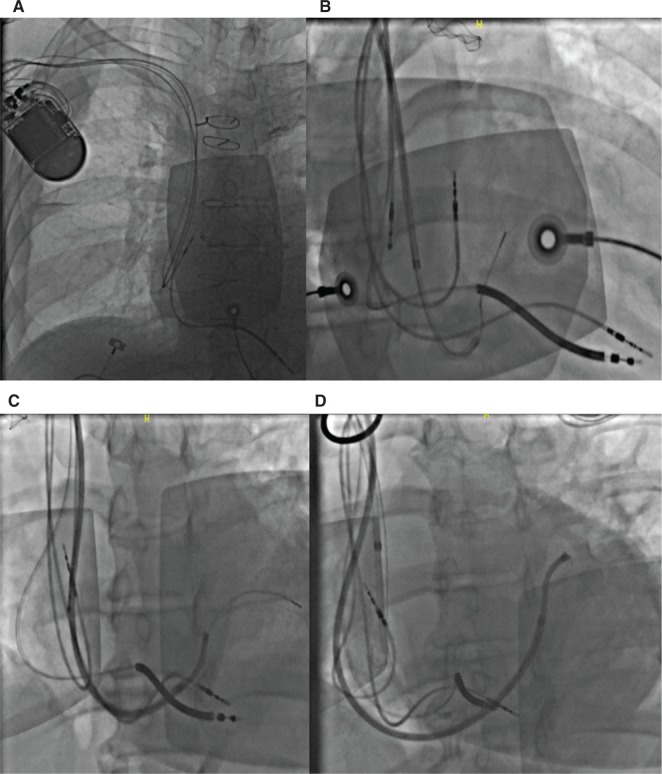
Right-sided CS cannulation. A: Chest X-ray showing acute angulation of the right subclavian–SVC junction. B: The outer sheath has a relatively straight course during CS cannulation. C: The outer sheath running along the lateral right atrial wall to facilitate advancement of the wire and the inner sheath into the CS. D: Optimal CS sheath position for successful lead delivery.
